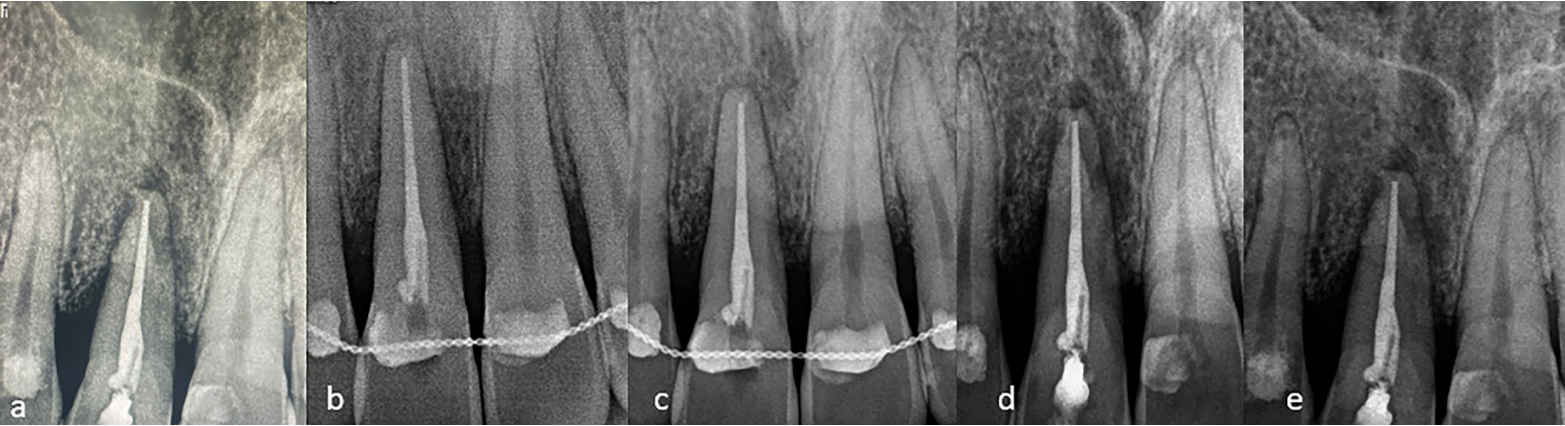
a) Radiographic image depicts- immediately after intentional re-implantation b) Radiograph after 6 months follow up c) Radiograph after 1 year follow up d) Radiograph after 2 years follow up e) Radiograph after 3 years.
